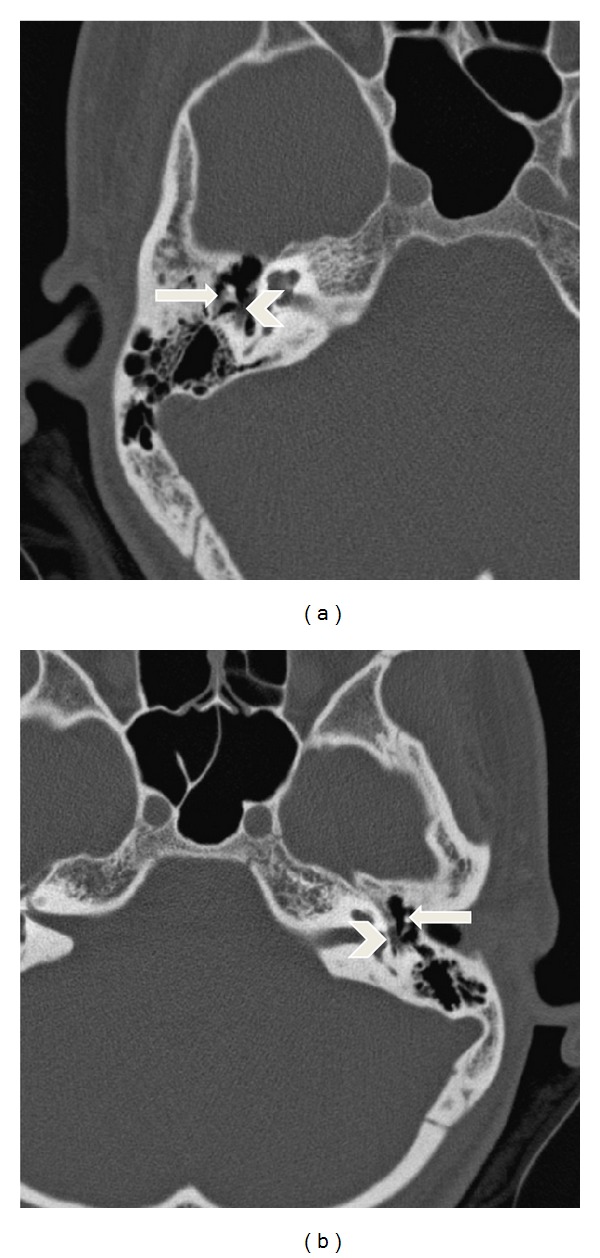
Right (a) and left (b) axial CT scan of the temporal bone showing the bilateral absence of the stapes (arrow), the abnormal position of the facial nerve (arrowhead), normal pneumatization of the mastoid air cells, and normal inner ear morphology.
